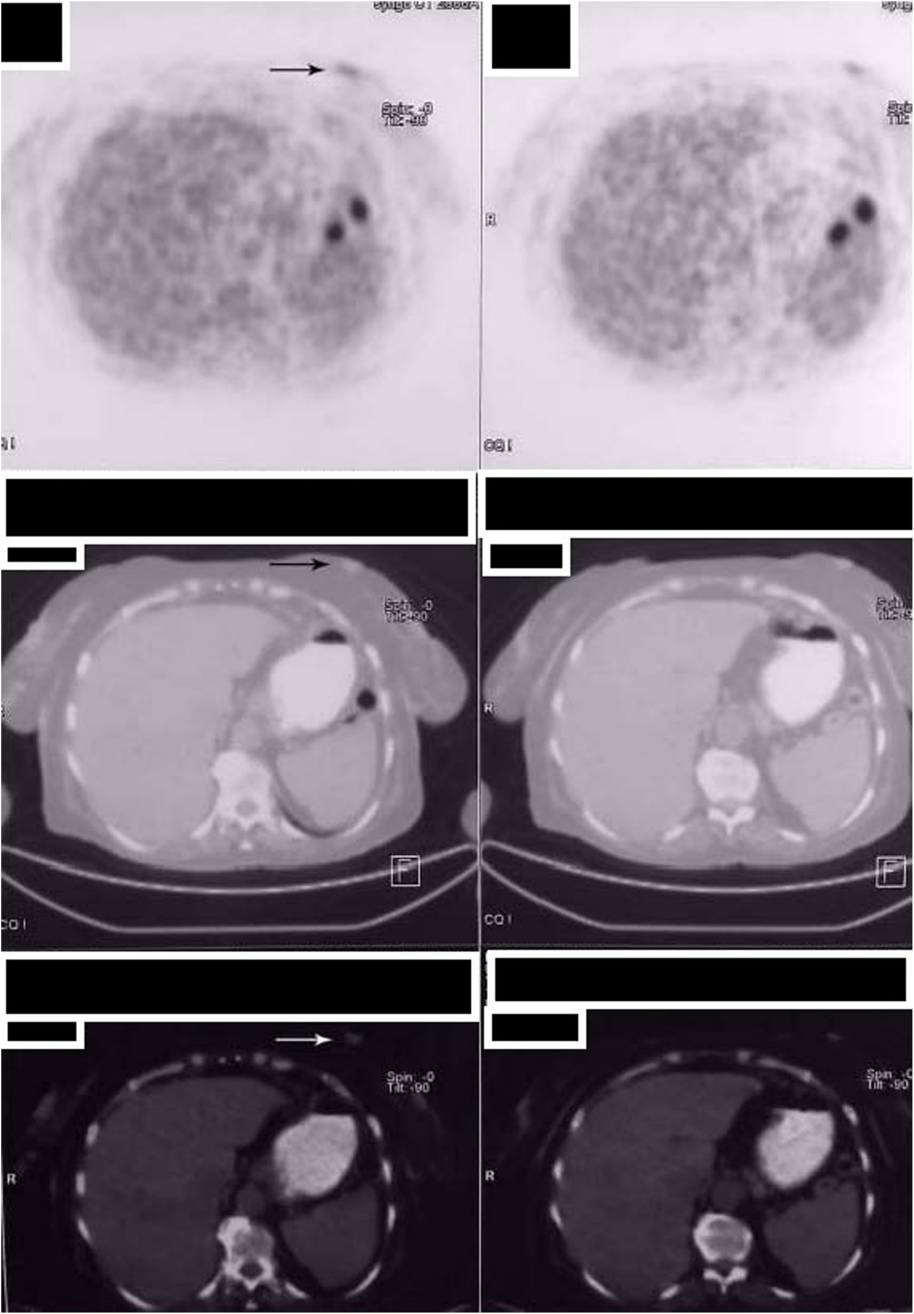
The positron emission tomography-computed tomography images showing hypermetabolic mass lesion in the lower inner quadrant of the left breast (selected arrows).
